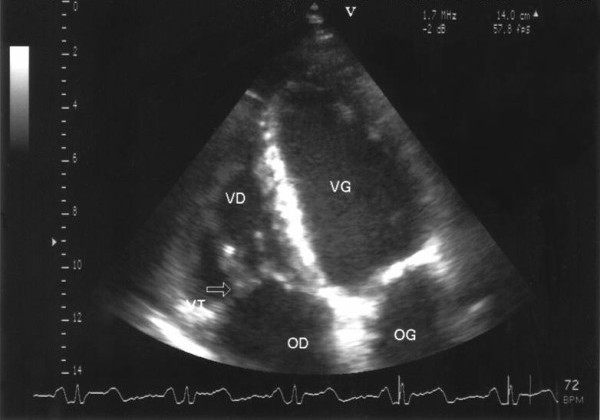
Transthoracic echocardiography after one year of treatment. No vegetation was found. The tricuspid valve is indicated by an arrow. VD; right ventricle: OD; right atrium: VG; left ventricle: OG; left atrium.
